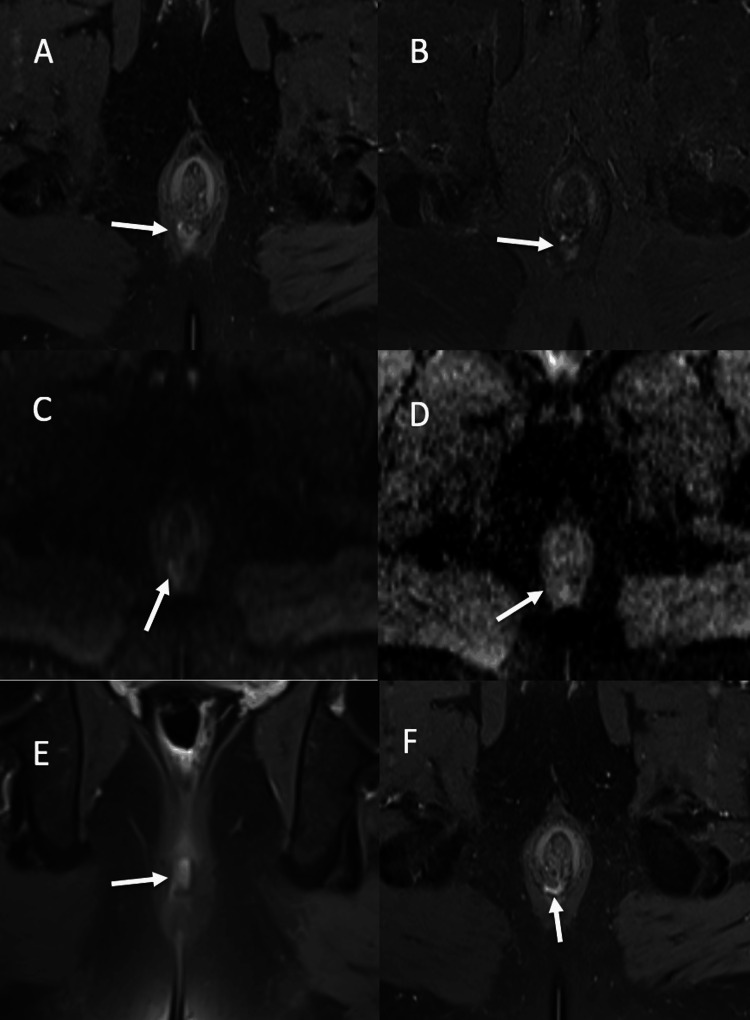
Perianal abscess in grade 2 intersphincteric fistula: (A-B) Axial post contrast fat suppressed T1-WI and axial STIR respectively, depicting a small abscess near internal opening of intersphincteric fistula showing heterogeneous enhancement on post contrast fat suppressed T1-WI (A) and hyperintense signal on STIR (B). (C-D) Axial DWI and ADC respectively, depicting abscess showing hyperintense signal on DWI (C) with corresponding signal drop on ADC (D). (E-F) Coronal and axial post contrast fat suppressed T1-WI respectively, showing intersphincteric fistula with internal opening at 6-o’clock position. T1-WI: T1 weighted image; STIR: short tau inversion recovery; DWI: diffusion weighted imaging, ADC: apparent diffusion coefficient
